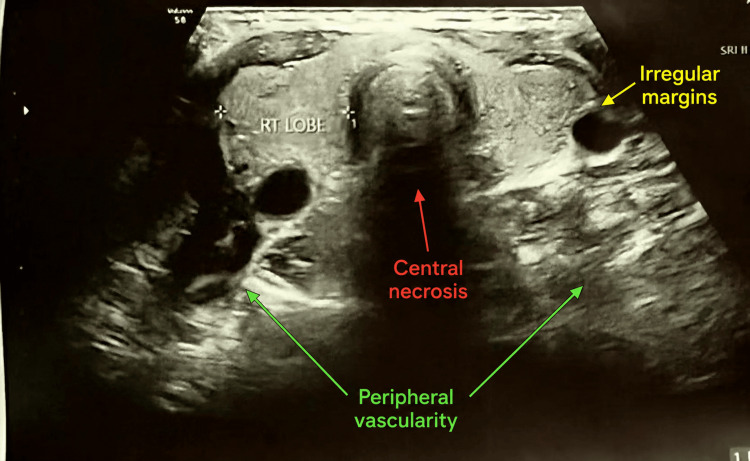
Ultrasound revealed a large, irregular, hypoechoic lesion in the right supraclavicular region.
